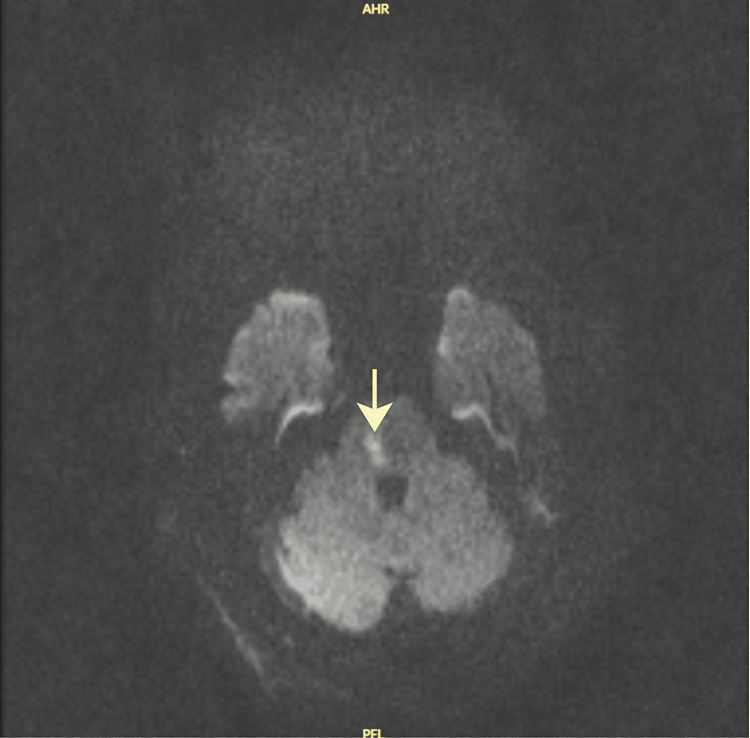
MRI of the head with diffusion-weighted imaging (DWI) sequence demonstrating a small infarct (yellow arrow) in the right pons
